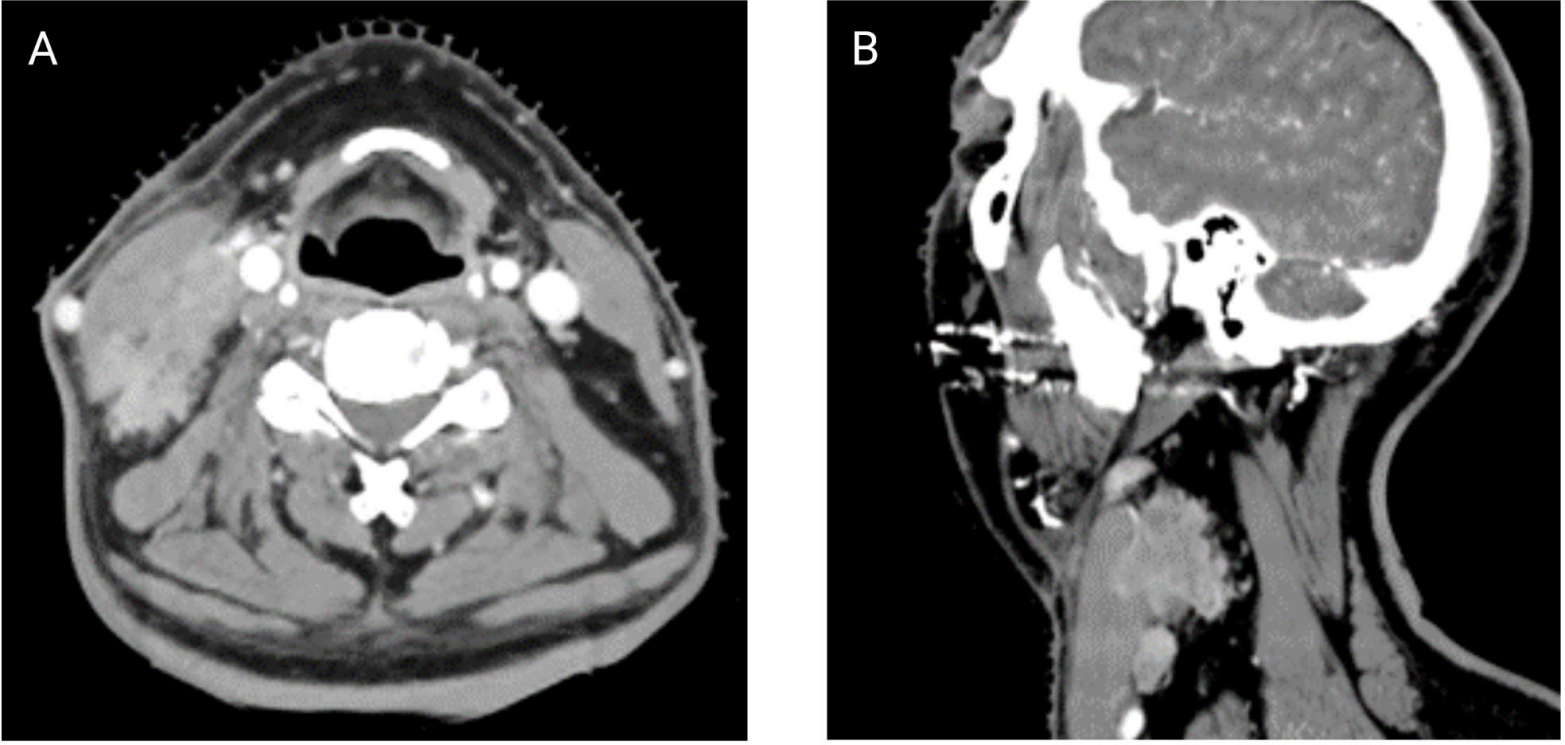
Contrasted axial (A) and sagittal (B) CT scans of a patient with clear ENE (Images kindly provided by Dr. Santiago Medrano).
